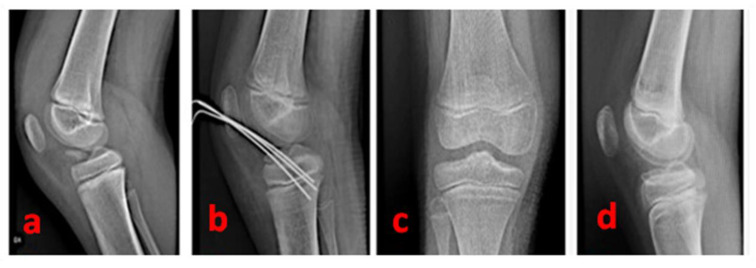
A radiographic summary overview of the right knee of a 9-year-old female patient—(a): pre-operative lateral view of a 3-type TSA; (b): post-operative radiographic control showing the cross-shaped geometry of the Kirschner wires; (c): antero-posterior radiographic control 15 months after surgery; (d): lateral radiographic control 15 months after surgery (complete restoration).
